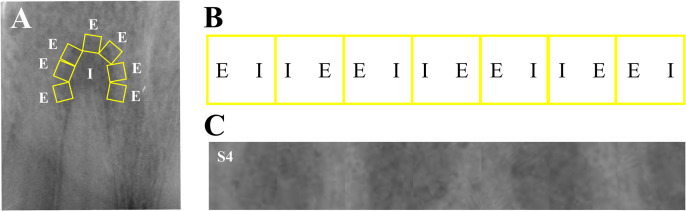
Representative example of the definition of ROIs S4. The grayscale gradient transition zone from lesion to surrounding tissue was totally covered with several squares (A). The radiograph was segmented according to the orientation of squares (B: I, the internal area of apical lesion; E, the external area of apical lesion). The segmented radiographic fragments were reorganized and reassembled, picture with light and dark vertical stripes was obtained (C).
